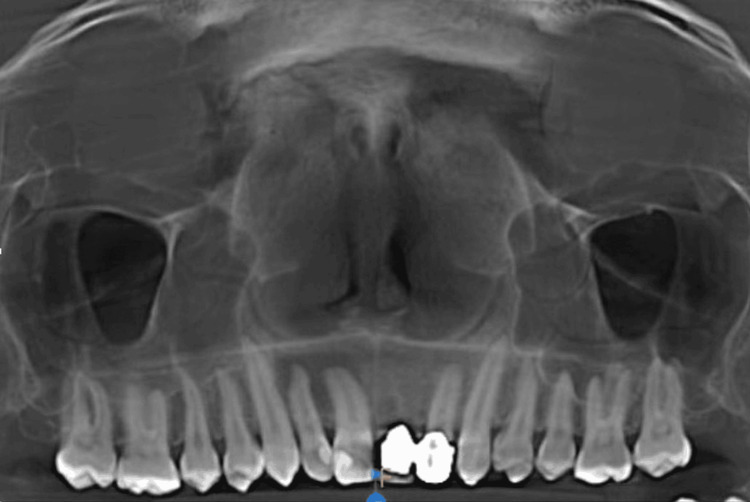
Mucosal thickening - OPG view OPG: Orthopantomogram
